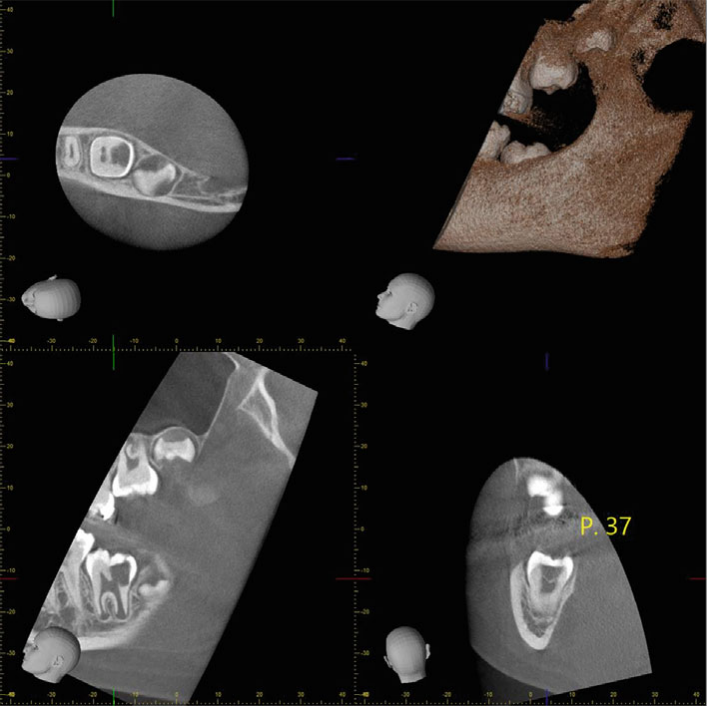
CBCT of Tooth 37: hypodense lesion extending to the pulp chamber, no periapical inflammation.
